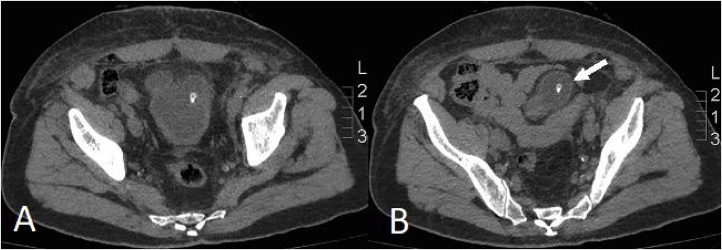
A plain Computed Tomography scan of the pelvis, showing the catheter to be located within the bladder (A), and advanced, with the tip seen within a bladder diverticulum (arrow), located at the left anterior bladder wall (B).
